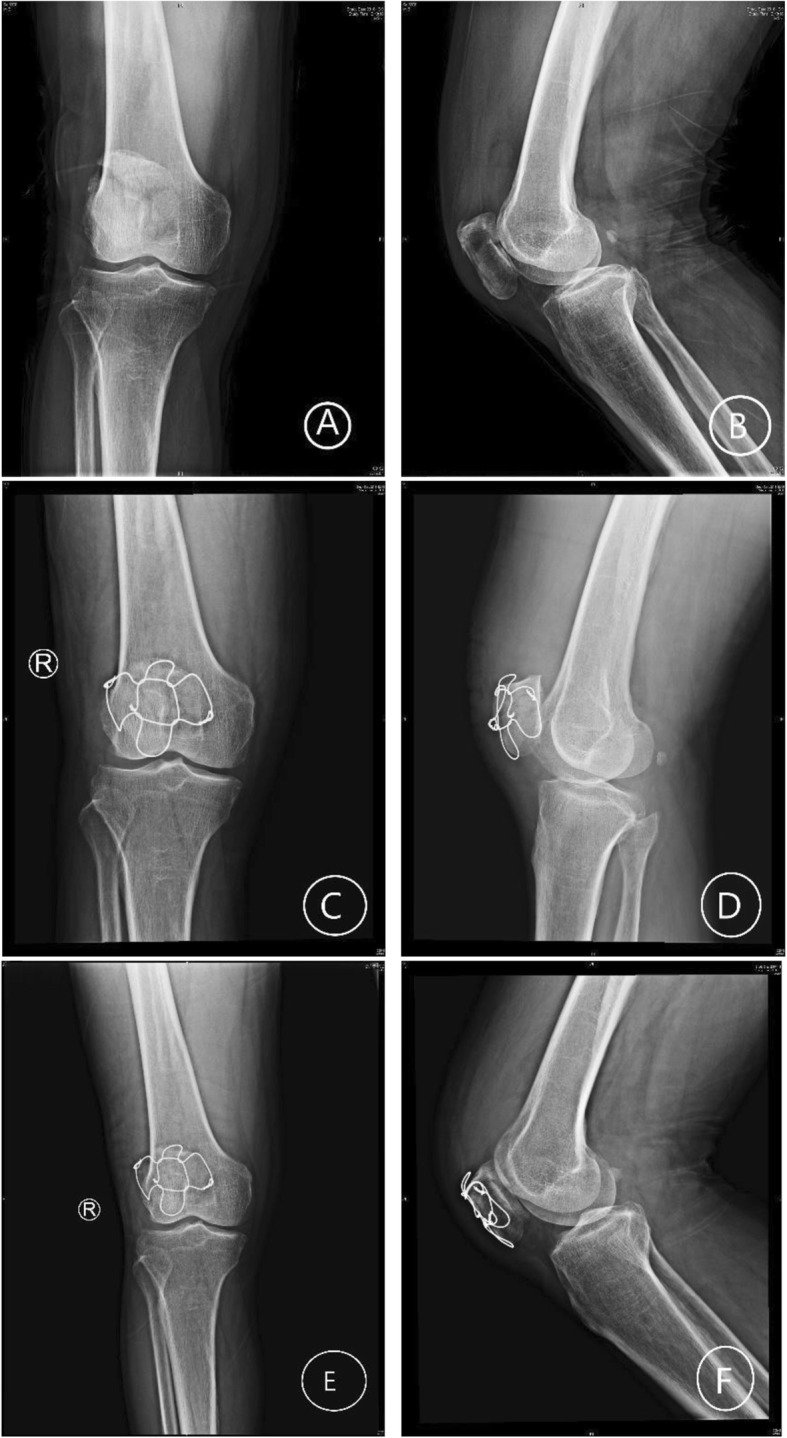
A 36-year-old female with severely comminuted patellar fracture (34-C3). a, b X-ray before surgery. c, d Post-fixation with modified cerclage wiring. e, f 3 months after surgery
